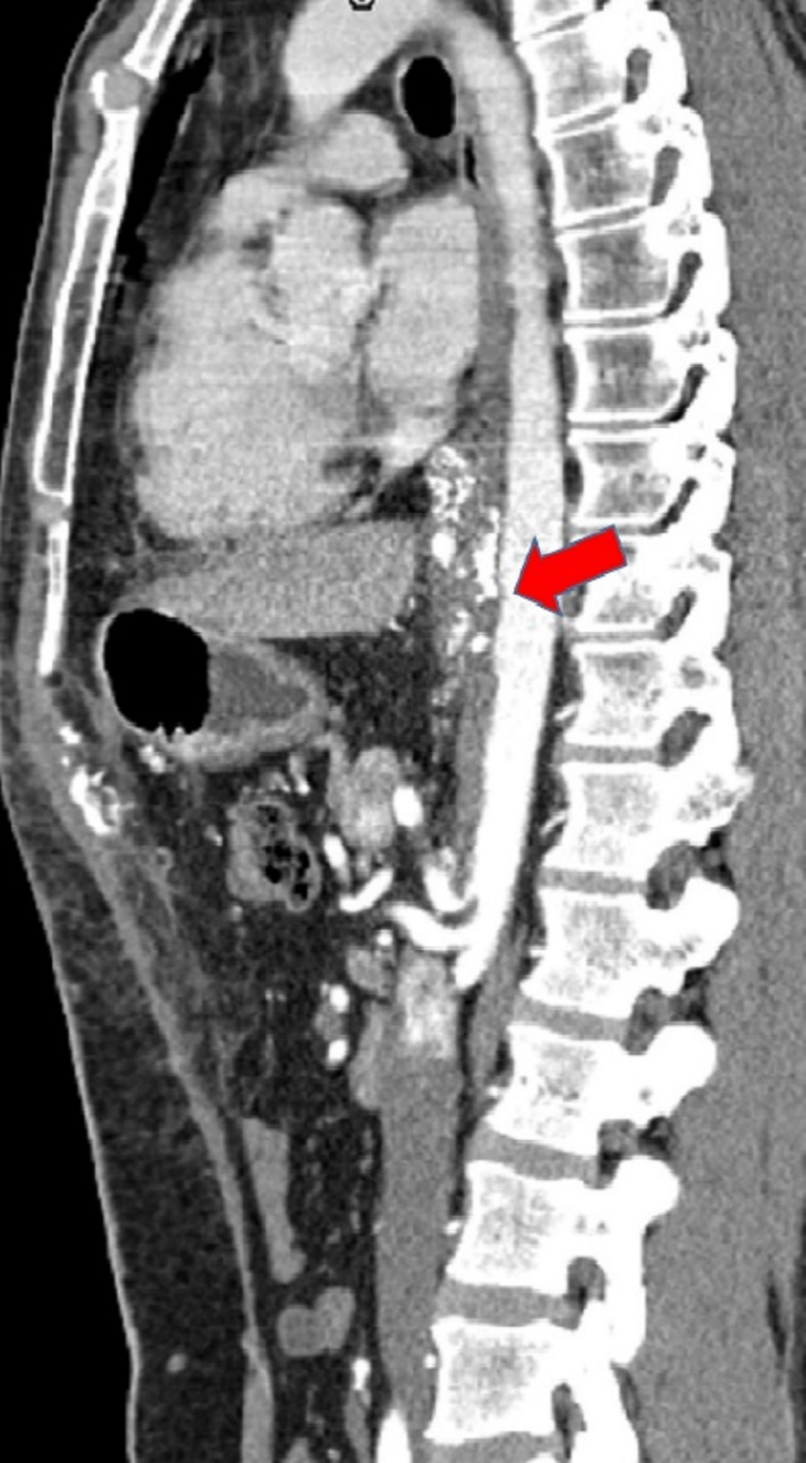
CT and MRI Preoperative Images Sagittal CT - Suspicious of aortic and celiac axis invasion (arrow) - loss of plane between tumor and descending aorta
